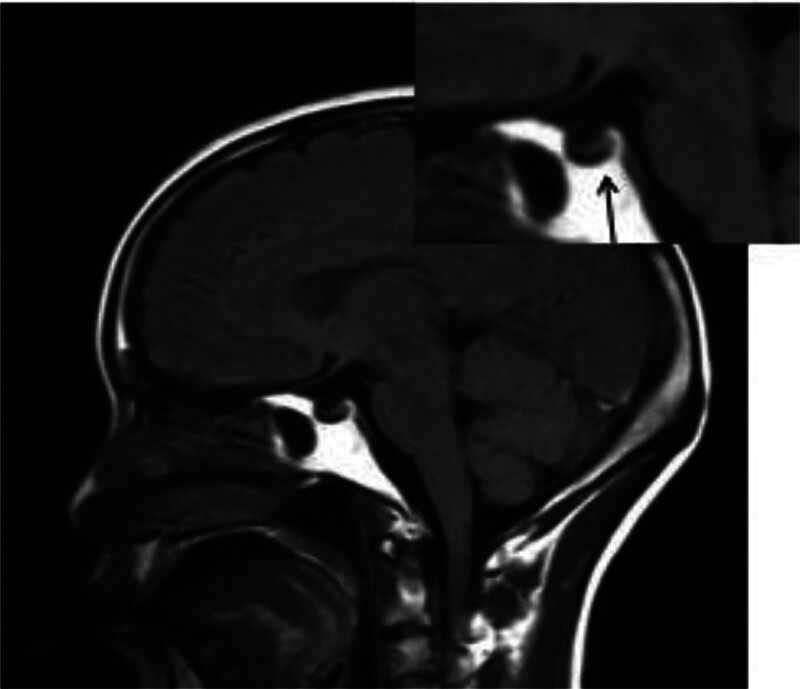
The sagittal MR images of patient 1 showing empty sella (arrow) secondary to postpartum pituitary necrosis. MR = magnetic resonance.
